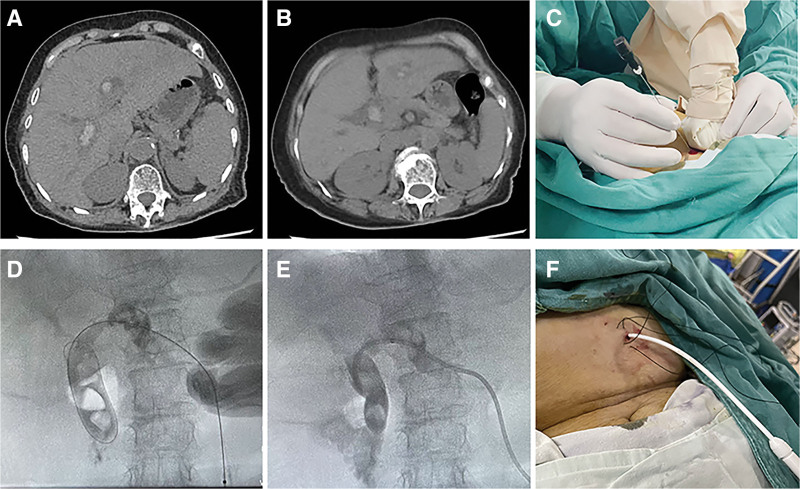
Preoperative abdominal CT scan confirming biliary stone distribution and anticipated puncture pathway in a 78-year-old female patient (A and B). Successful intraoperative placement of an 8.5 Fr drainage catheter into the common bile duct under combined ultrasound and cholangiography guidance (C–F). CT = computed tomography.
